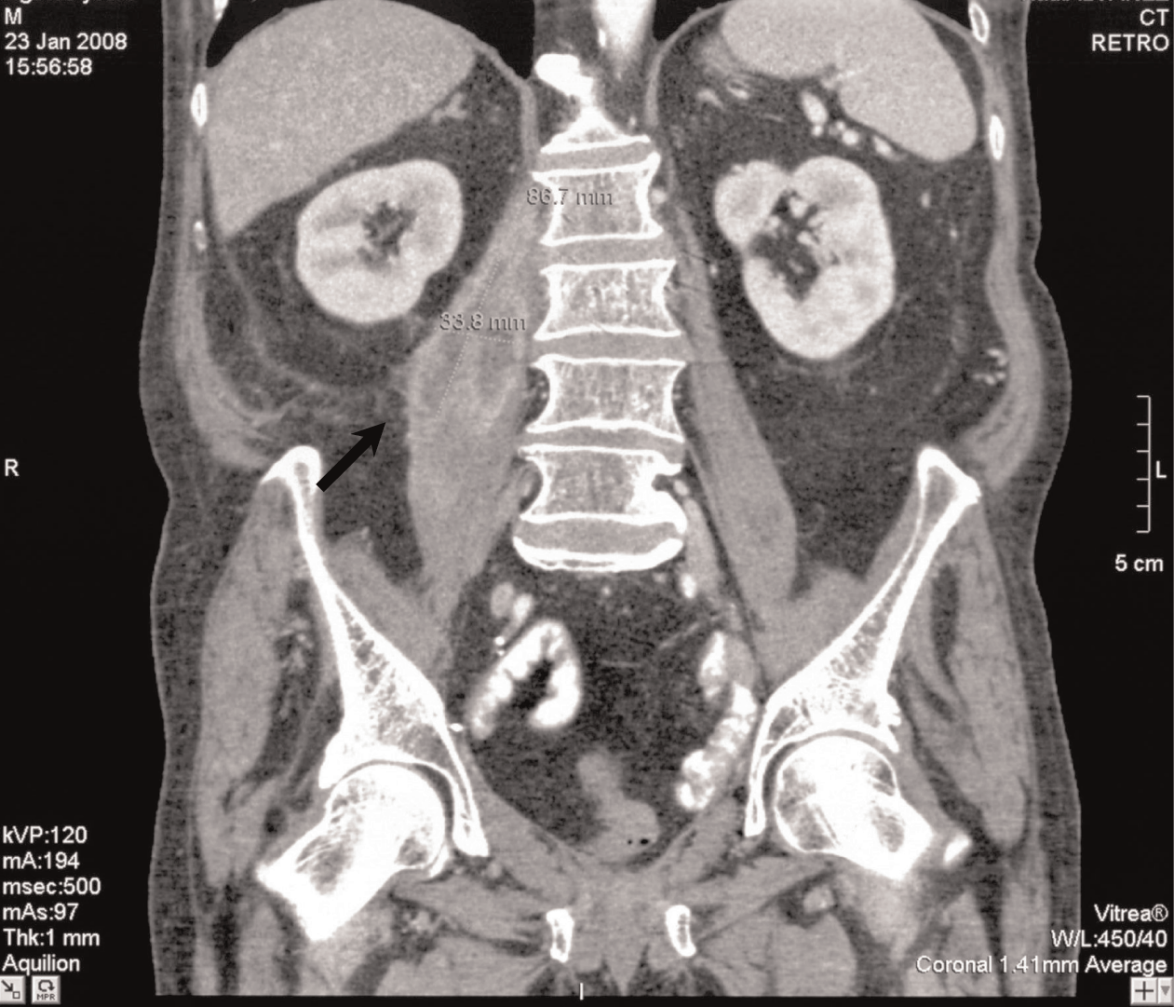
Computed tomography scan showing right psoas abscess.
